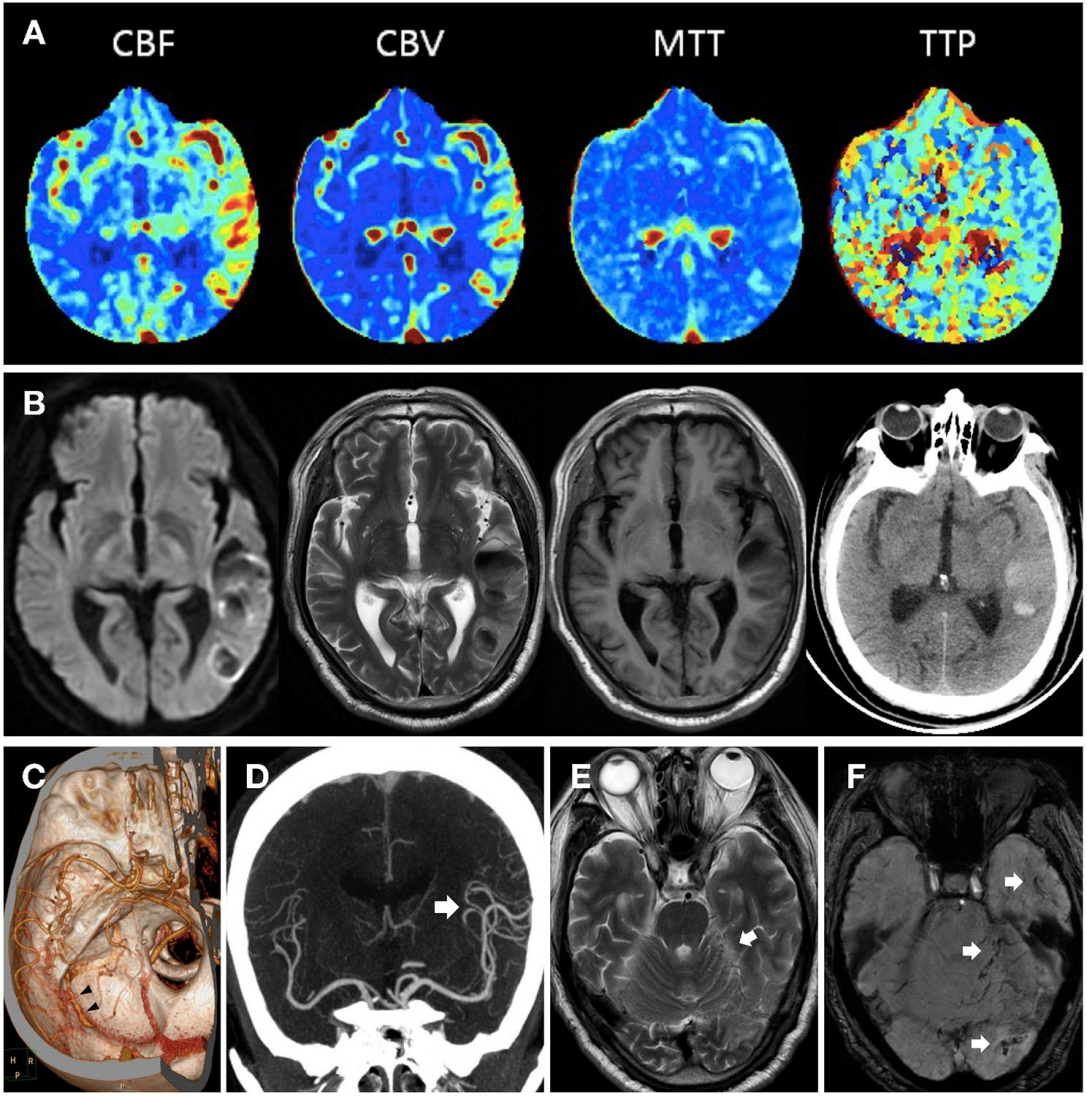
Cranial imaging appearance of the patients. (A) CT perfusion showed regional hyperperfusion in the left temporal lobe, with characteristics of a significant increase in cerebral blood flow (CBF), a mild increase in cerebral blood volume (CBV), and a prolonged mean transit time (MTT) and time to peak (TTP). (B) Cranial MRI and CT indicated hemorrhagic transformation in the left temporal lobe after intravenous thrombolysis. (C) Cranial CTA showed an early visualized left transverse sinus segment (black arrowhead), which was previously ignored and misinterpreted as venous contamination. (D) Hypervascularity in the left cerebral hemisphere (white arrow) was noted in cranial CTA, consistent with the regional hyperperfusion pattern. (E) Cranial MRI T2 sequence revealed tortuous abnormal vascular flow voids (white arrow) in the left temporo-occipital lobe and cerebellum. (F) Cranial susceptibility-weighted imaging showed clusters of engorgement veins, thought to represent collateral venous flow (white arrow)
